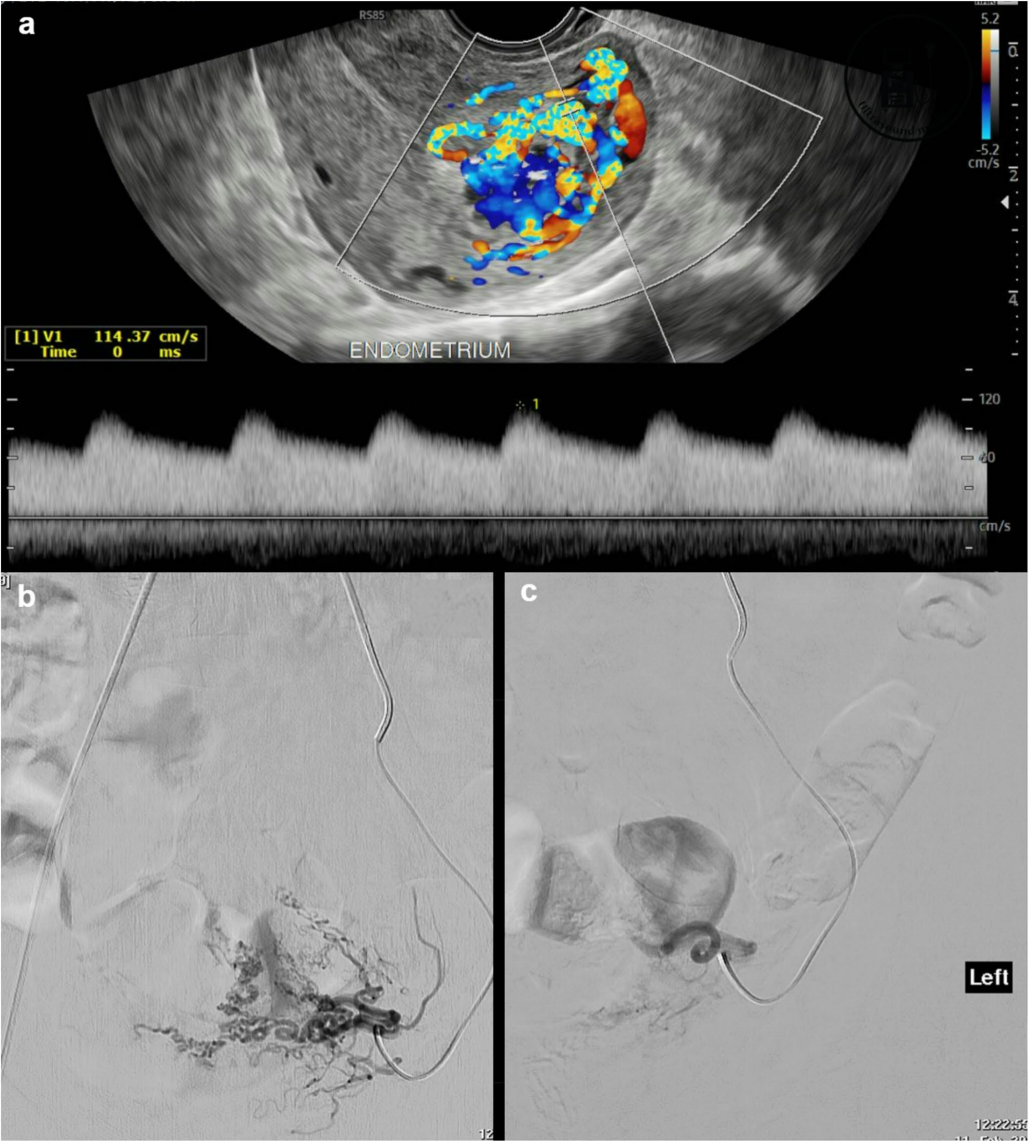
A 37-year-old female presented with vaginal bleeding after miscarriage. Pelvic ultrasound (a) confirmed the UAVM. Selective left uterine artery angiogram with a micro-catheter confirmed a cluster of tortuous and hypertrophied abnormal vessels (b), confirming the ultrasound finding. Successful UAVM embolisation with absorbable haemostatic gelatin was achieved (c)
